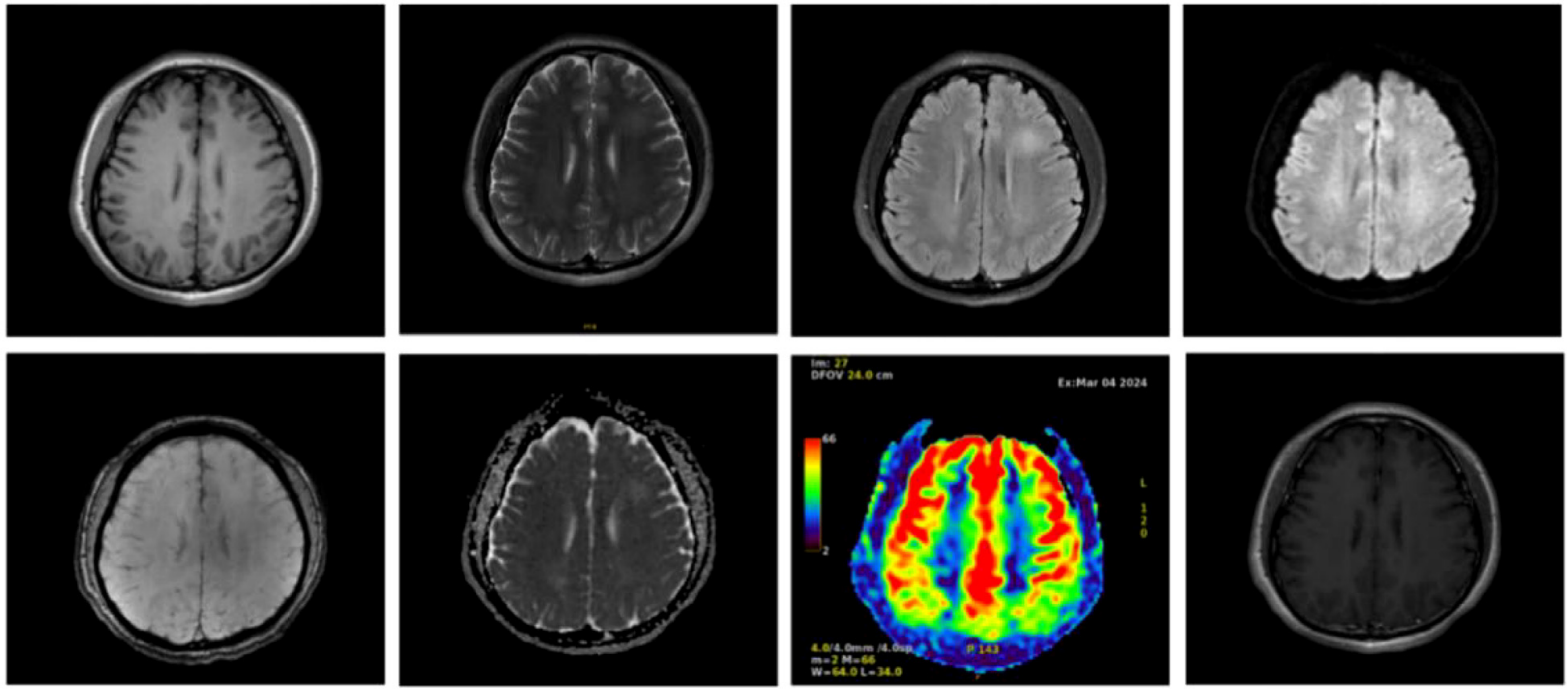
Cranial magnetic resonance imaging of the patient with autoimmune encephalitis showing a frontal lobe lesion, no abnormal low-signal foci in SWAN, and no significant abnormalities in blood flow in the lesion area in ASL.
